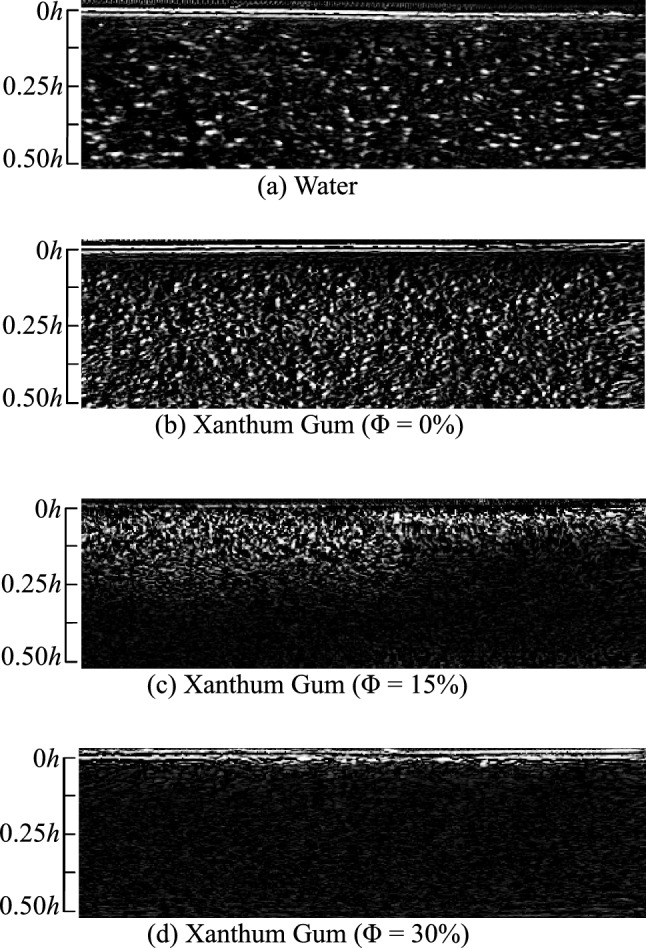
Ultrasound images taken near the pipe wall (top) for a water b XG$${\Phi }0\%$$, c XG$${\Phi }15\%$$ and d XG$${\Phi }30\%$$. The pipe wall is located along the top edge and flow is from left to right. A scale is provided in each image indicating depth into the pipe
